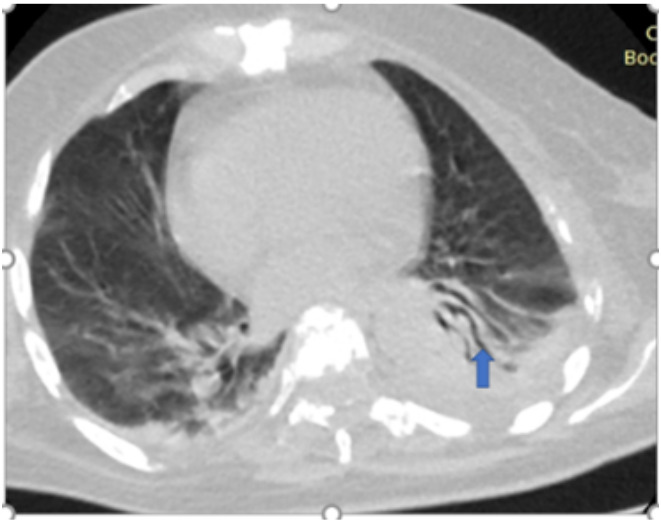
57- year -old female, breast cancer patient had fever, chest pain and cough for 9 days, Axial non-contrast enhanced CT Scan of the chest, lung window showed left segmental consolidation with air bronchograms (Arrow), minimal pleural effusion and minimal consolidation in the right lower lung zone. No peripheral distribution. Categorised as Atypical. Also noted is lytic destruction (metastatic) of the thoracic vertebral body. RT-PCR was positive, but the imaging features are not Typical and could represent pneumonia related to COVID-19 or a secondary infectious process.
